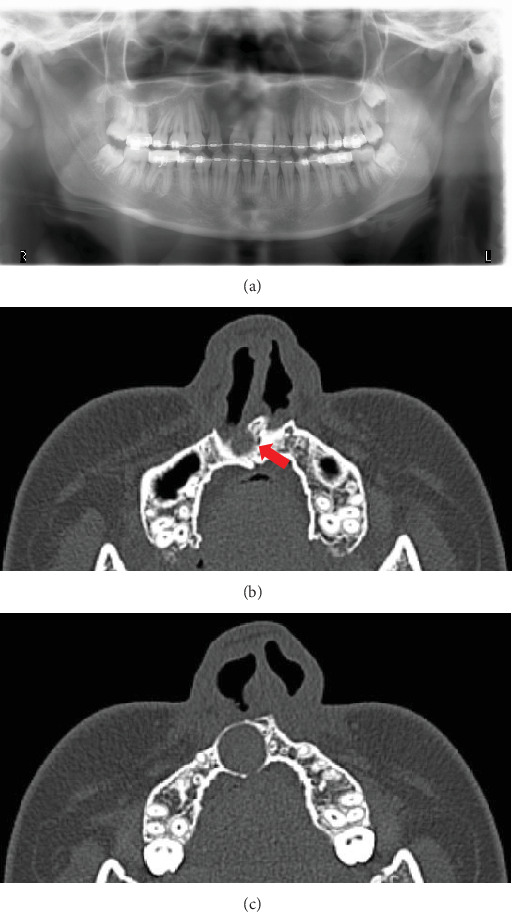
(a) Preoperative panoramic radiography and (b,c) CT. Continuity of the lesion with the incisive canal is confirmed (b, arrow).
